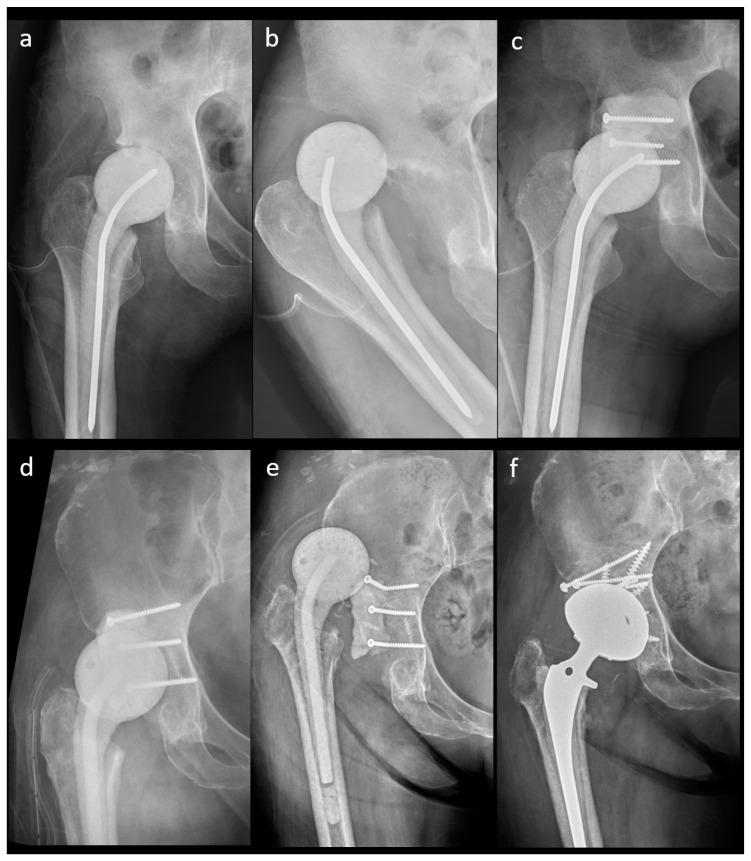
A 41-year-old female patient with an n-MS spacer: (a) post-operative radiographs, (b) posterior dislocation 2 weeks after spacer insertion, and (c) open reduction combined with temporary cement tectoplasty. A 68-year-old male patient with an n-MS spacer: (d) open reduction combined with tectoplasty after posterior dislocation 4 weeks after spacer insertion, (e) recurrent dislocation after tectoplasty, and (f) acute reimplantation with bone grafting and cemented stem after the dislocation event and free of complications.
